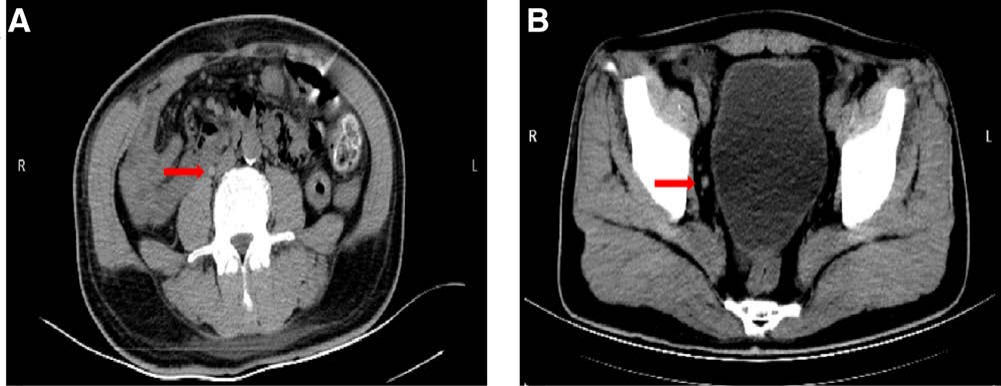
(A, B) The CT scan suggests that a blood clot in the right ureter. CT = computed tomography.
